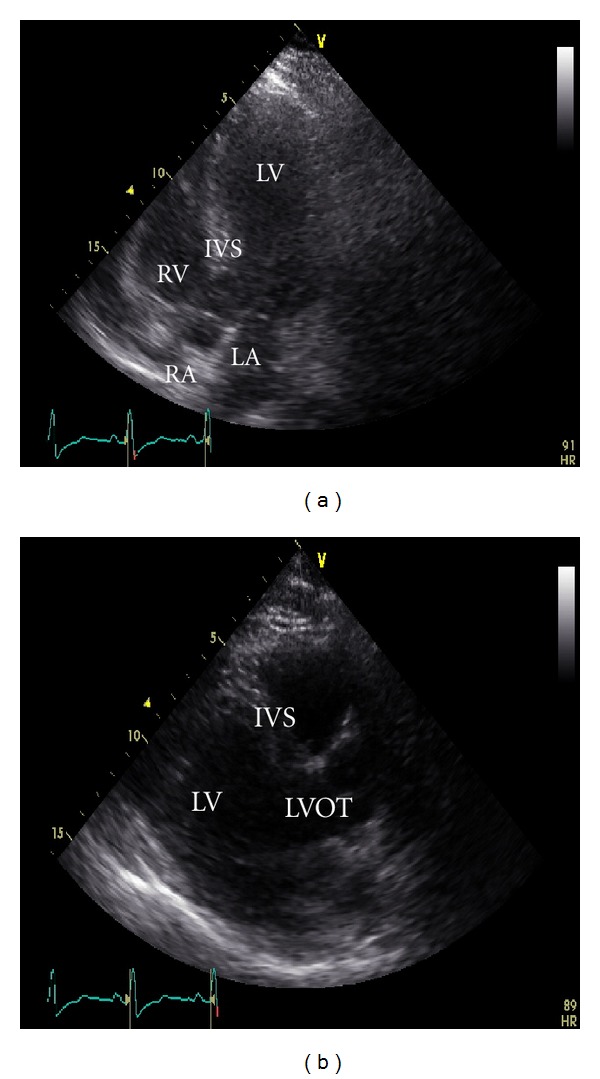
Apical four-chamber view (a) and parasternal long axis view (b) with poor acoustic window revealed thickened left ventricular walls. LV: left ventricle; LA: left atrium; LVOT: left ventricular outflow tract; RV: right ventricle; RA: right atrium; IVS: interventricular septum.
